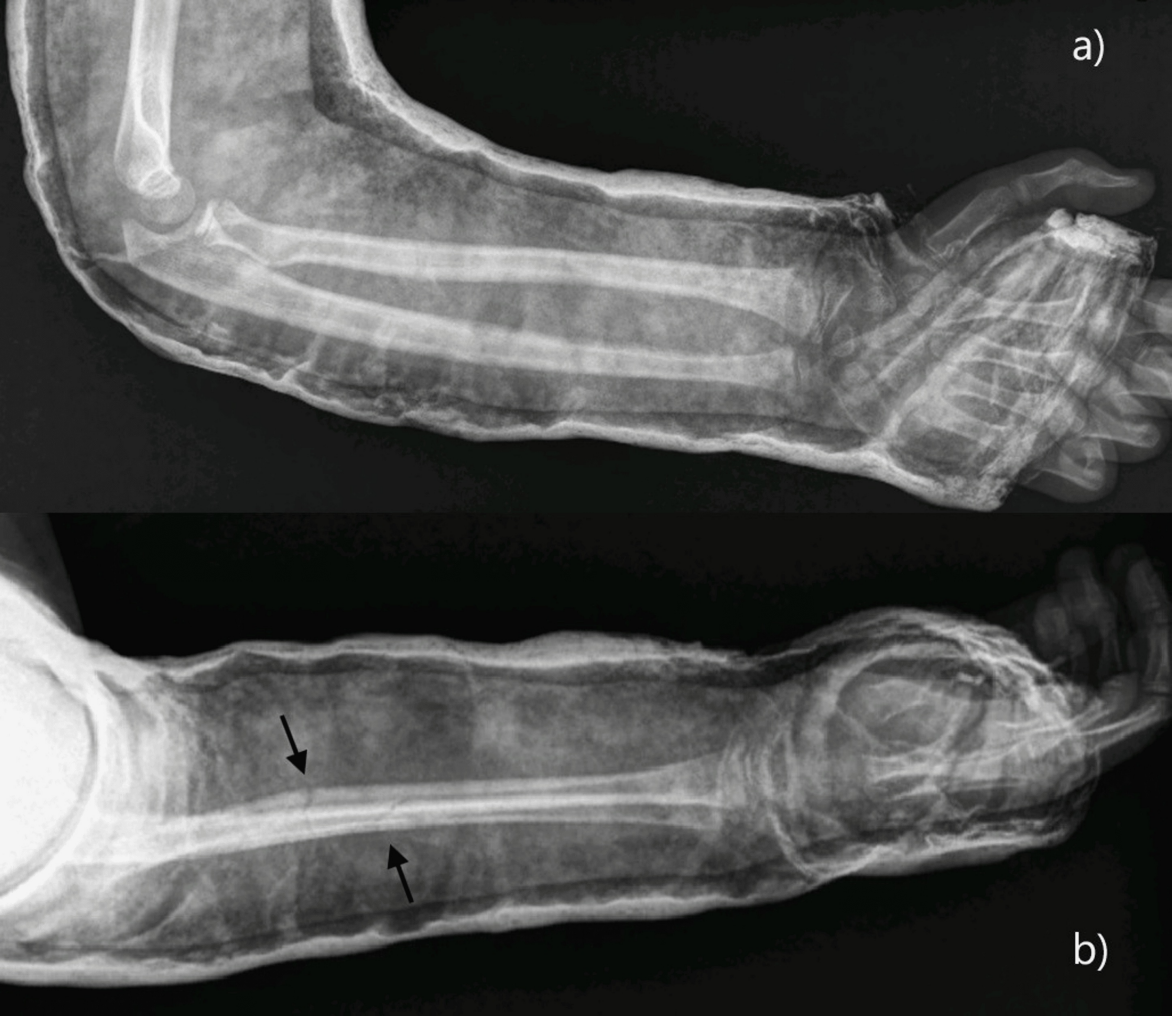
Forearm radiograph post-closed reduction a) Anteroposterior view; b) side view.
